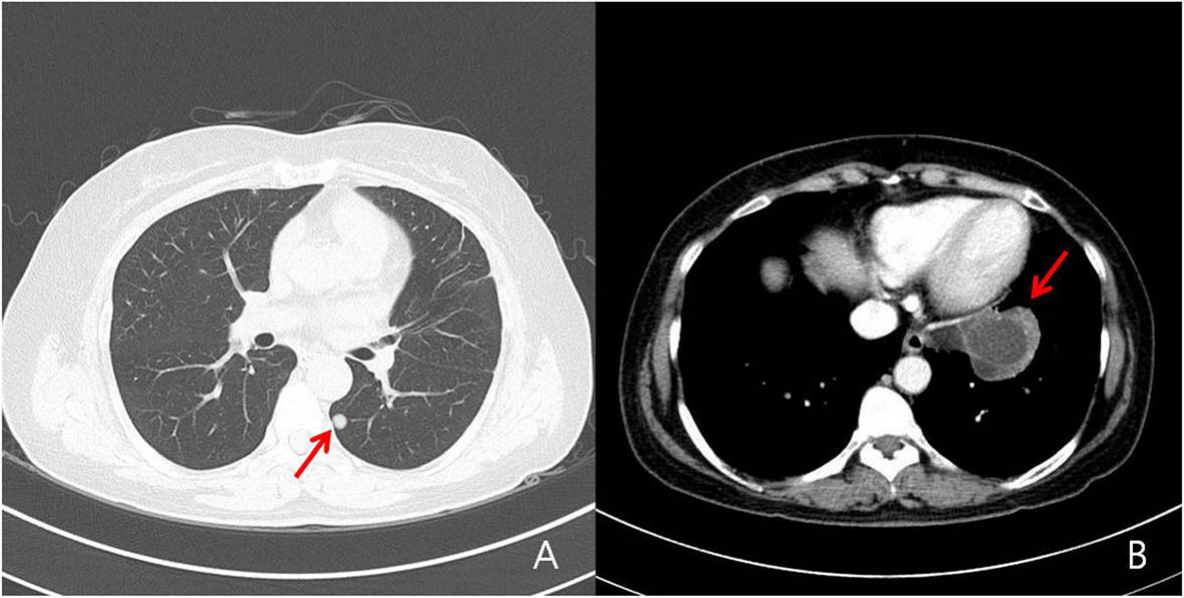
Chest CT showed cystic lesion involving LUL (arrow) (B) and well-defined mass involving LLL (arrow) (A).
